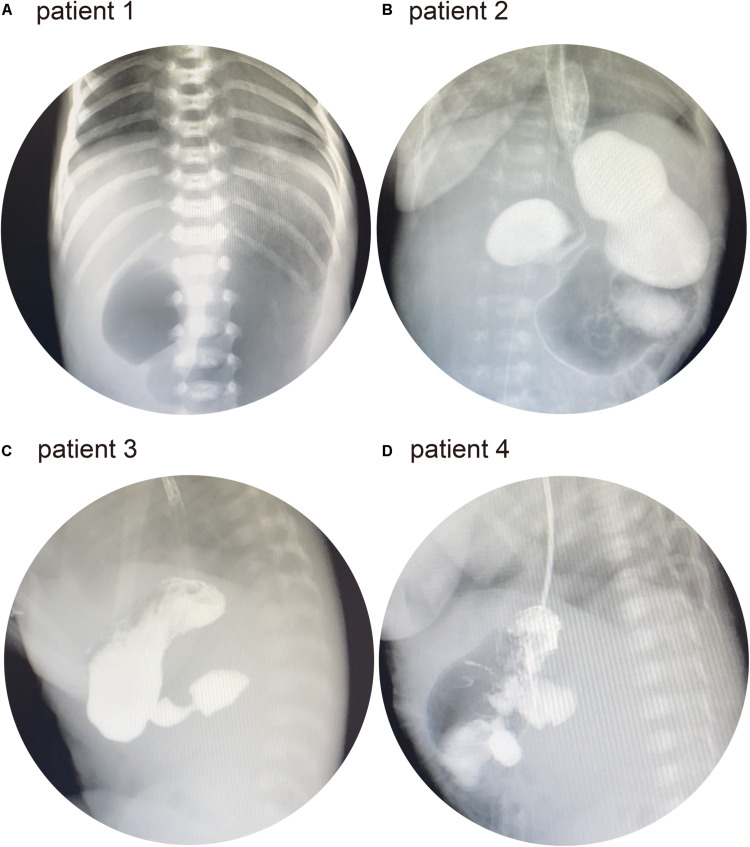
(A) Patient 1: abdominal X-ray showed the “double bubble” sign. (B) Patient 2: upper gastrointestinal series (UGI) showed distended stomach and duodenal bulb. (C) Patient 3: UGI showed a distended duodenal bulb. (D) Patient 4: UGI showed a distended duodenal bulb.
